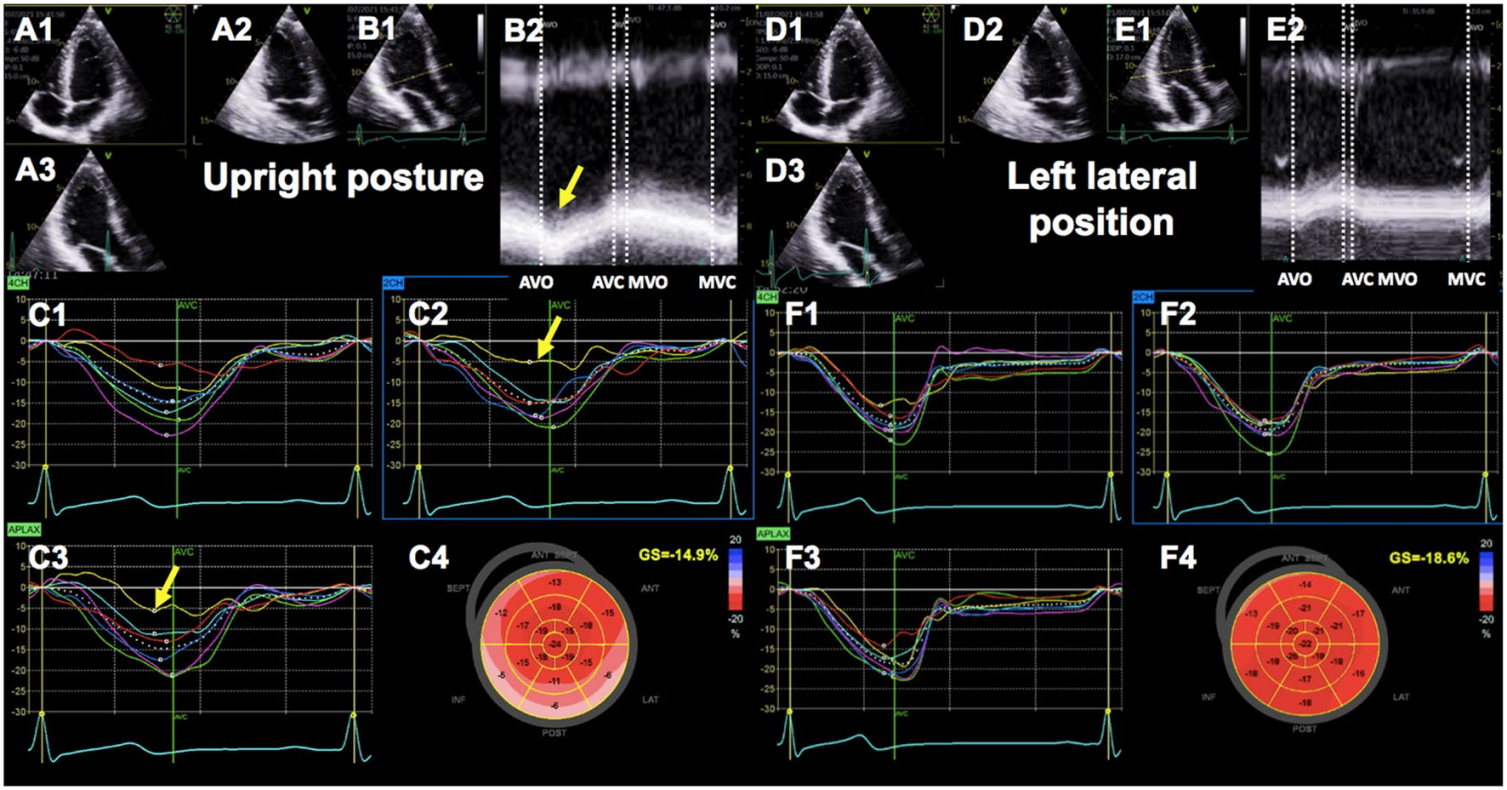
Global longitudinal strain measured by 2D echocardiography in upright (A-C) and left lateral position in the same athlete (D-F). Apical long axis, 2- and 4-chamber view (A1-A3, D1-D3) with anatomical M-Mode in the apical long axis view (B1-B2, E1-E2). Strain curves of all three apical views (C1-C3, F1-F3) with the corresponding bull’s eye (C4, F4). The yellow arrow indicates changes in left ventricular wall motion in comparison to left lateral position. *AVO *Aortic valve opening, *AVC *Aortic valve closing, *MVO *Mitral valve opening, *MVC *Mitral valve closing
